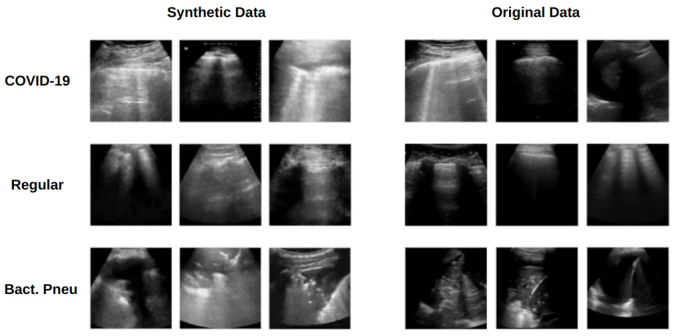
Comparison of original and synthetic ultrasound images generated using the WGAN-GP models.
